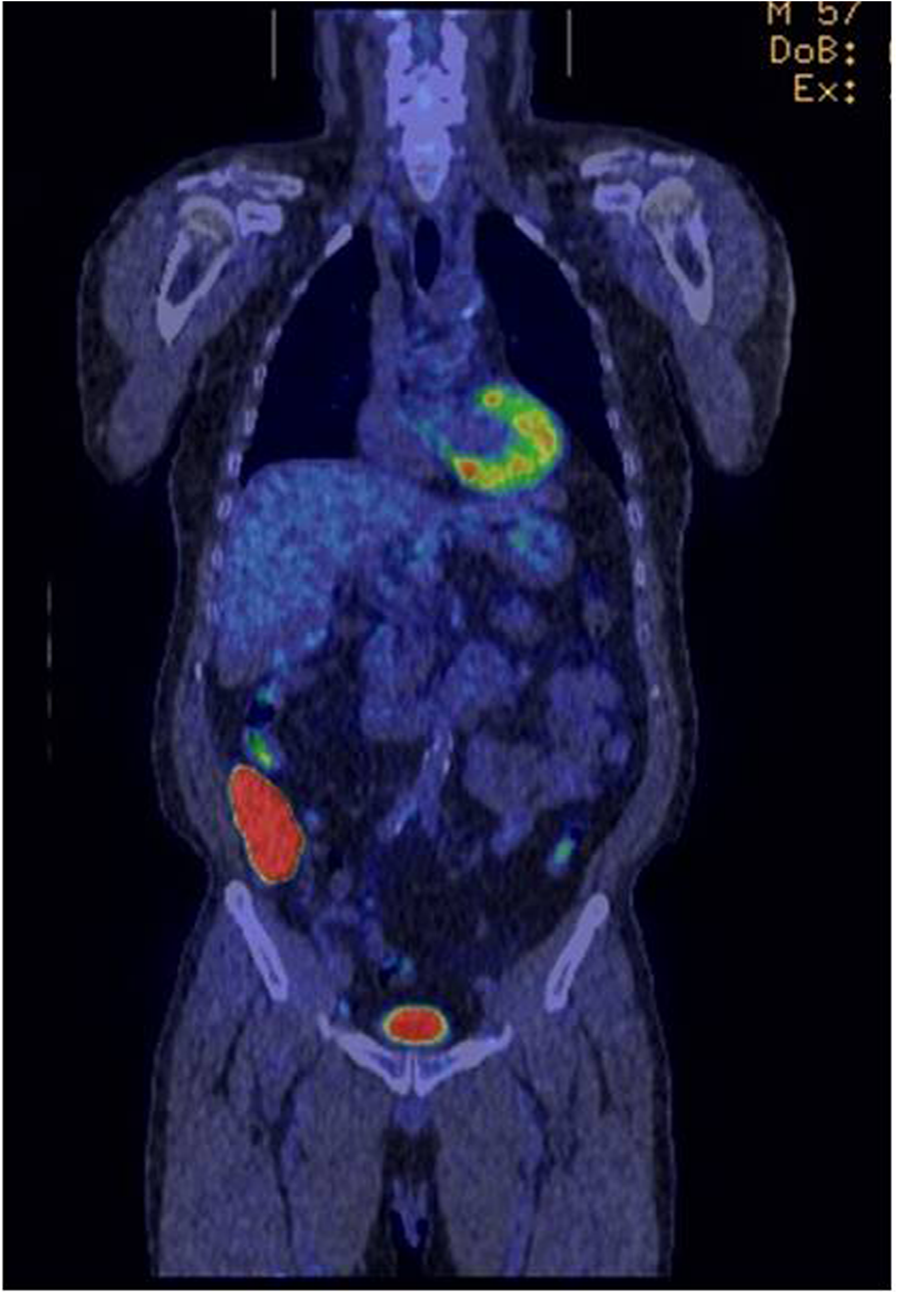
FDG-PET showing abnormal accumulation (SUVmax 41) in the abdominal wall
